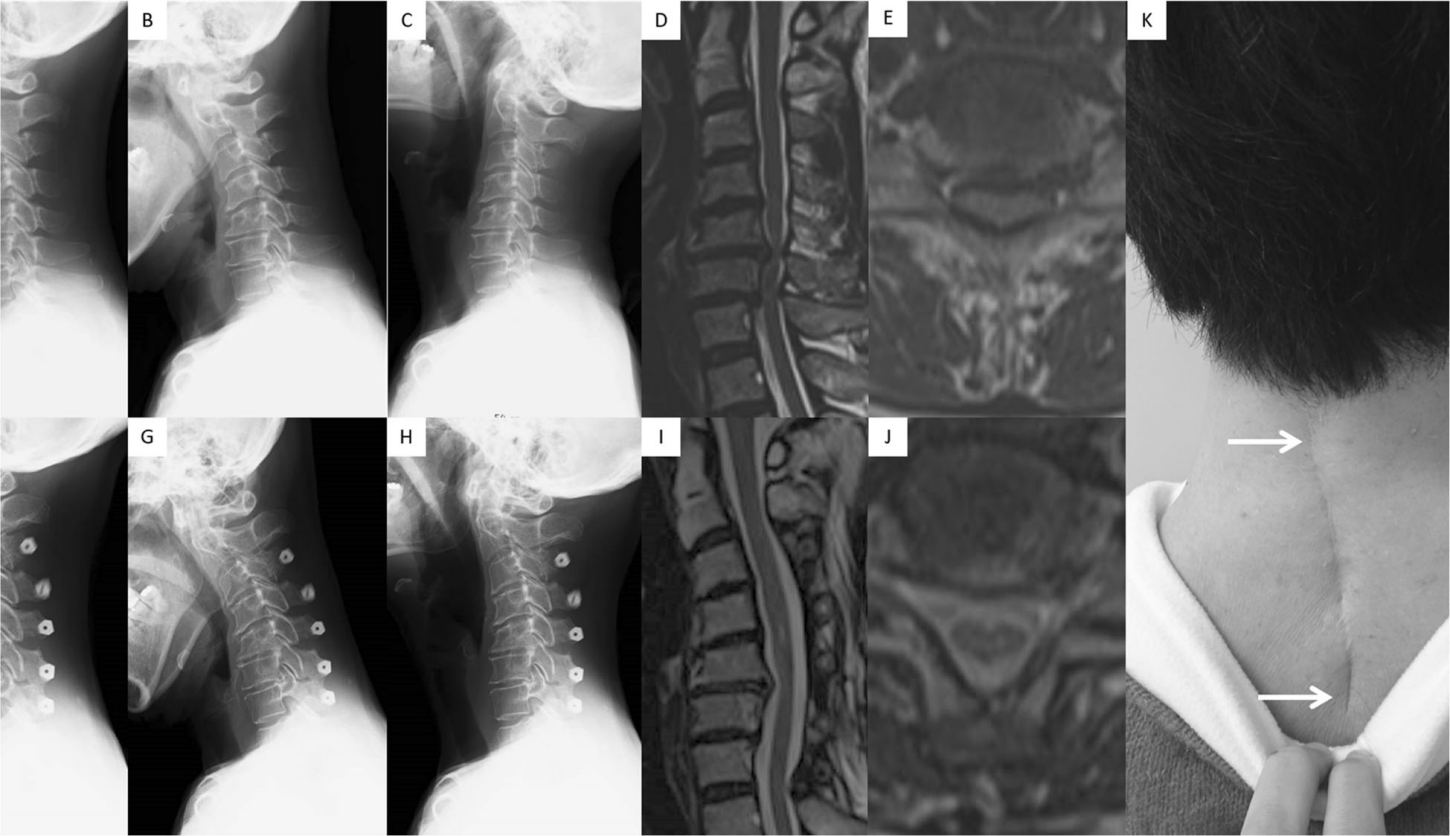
A 62-year-old woman presented with two-level spinal cord compression at C5/6 and C6/7 and underwent cervical laminoplasty (LP) from C3 to C7. a, b, c Preoperative lateral X-ray in neutral (a), flexion (b), and extension (c) positions. d, e Preoperative sagittal (d) and axial (e) images on T2-weighted magnetic resonance imaging (MRI). f, g,h Postoperative (at 28 months) lateral X-ray in neutral (f), flexion (g), and extension (h) positions. i, j Postoperative sagittal (i) and axial (j) images on T2-weighted MRI. K, Clinical photograph showing the operative scar (arrows). Pre−/postoperative C2/7 Cobb angles, range of motion, spinal cord diameter, and degree of posterior spinal cord shifting were 0/− 10 degrees, 56/19 degrees, 3.6/5.7 mm, and 3.8 mm, respectively. The pre- and postoperative Japanese Orthopedic Association (JOA) scores were 10 and 13. This patient showed progressive kyphosis postoperatively and complained of neck pain (numerical rating scale score of 7)
